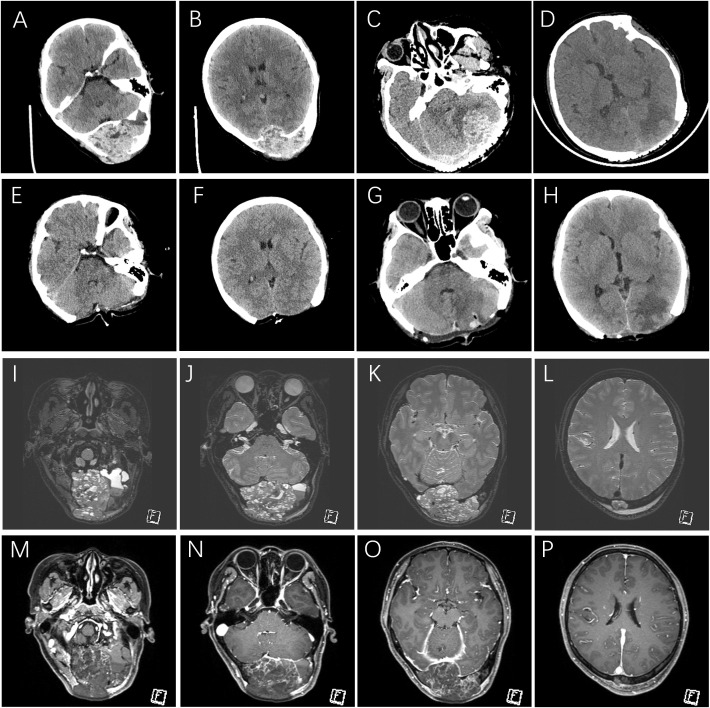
Serial computed tomography (CT) imaging of primary and recurrent occipital aneurysmal bone cyst and preoperative magnetic resonance imaging (MRI) characteristics. (A–H) axial CT images. (A, B) the lesion prior to the first surgery; (E, F) postoperative images after the first surgery; (C, D) recurrent disease following calvarial reconstruction; (G, H) postoperative images following resection of the recurrent lesion. (I–P) preoperative MRI of the initial presentation; (I–L) T2-weighted images; (M–P): contrast-enhanced T1-weighted sequences.
